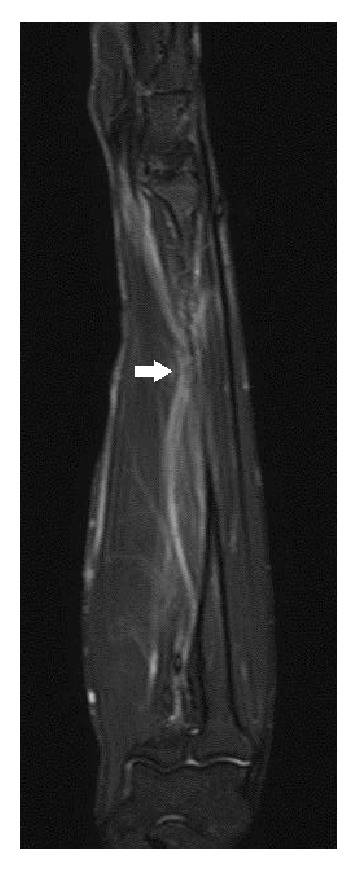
Short tau inversion recovery magnetic resonance imaging shows significant deviation of the median nerve toward the fracture site of the radius (arrow).
